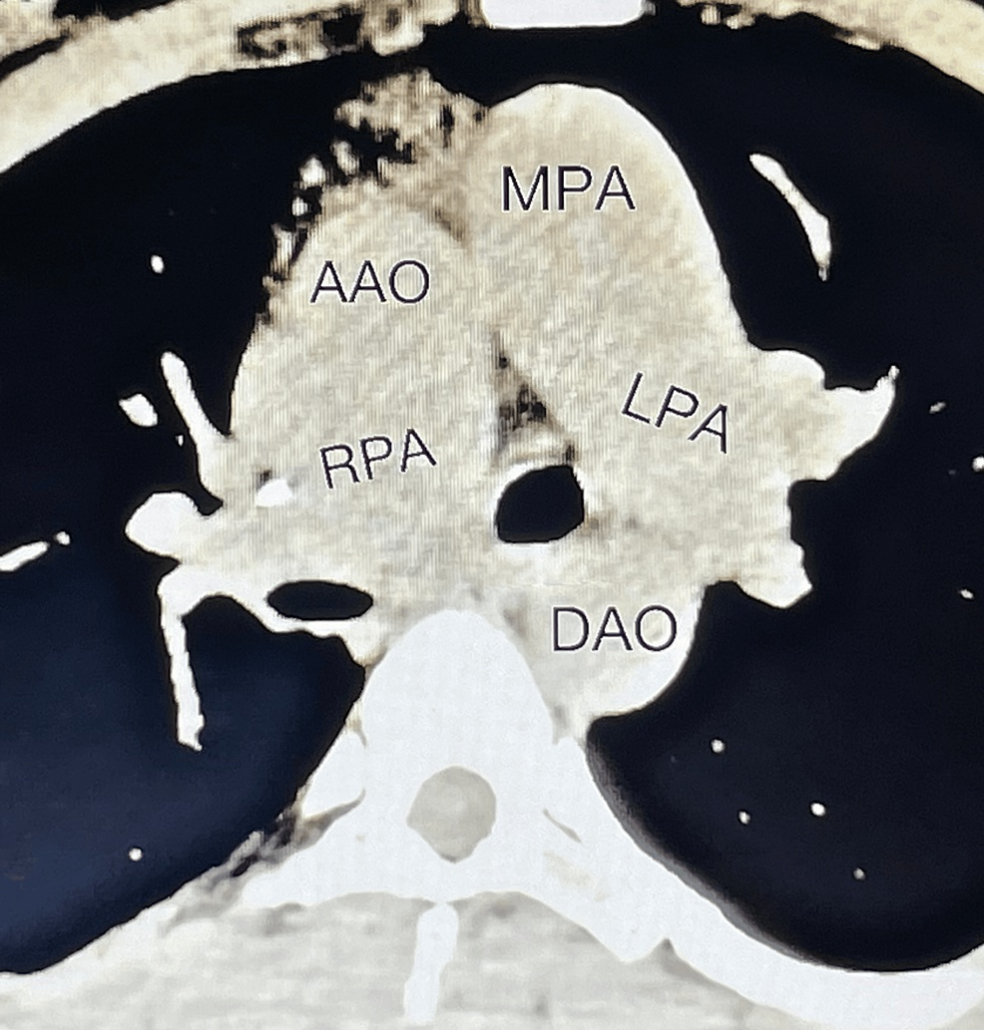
Chest CT scan PDA and an abnormal origin of the right pulmonary artery from the right ascending aorta. AAO: ascending aorta, RPA: right pulmonary artery, DAO: descending aorta, LPA: left pulmonary artery, LV: left ventricle, MPA: main pulmonary artery
